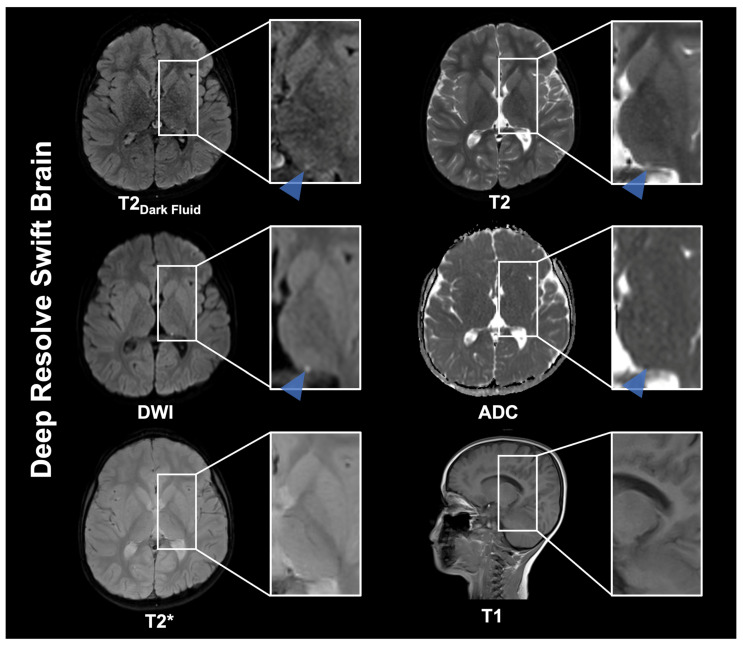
Presents additional images from the Deep Resolve Swift Brain protocol in the same 4-year-old boy, who developed a left vertebral artery dissection after neck trauma, resulting in a posterior circulation stroke. The protocol includes axial T2-weighted dark-fluid, T2-weighted, diffusion-weighted, and T2*-weighted sequences, as well as a sagittal T1-weighted sequence. A T2 well-defined punctate ischemic lesion in the left posterior thalamus is evident on diffusion-weighted images with a corresponding hypointense signal on the ADC map.
